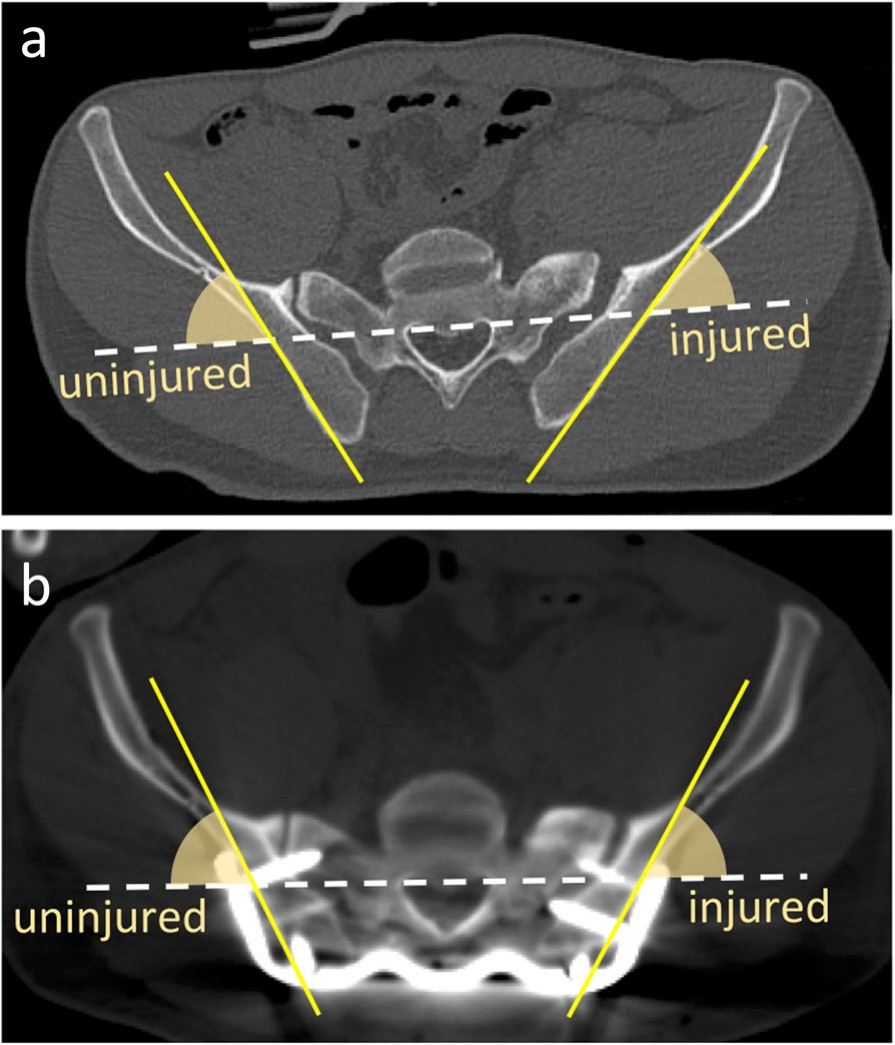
a Pre- and b Post-operative CT evaluation. The angle between the horizontal plane of the sacrum (white dotted line) and the lateral surface of the ilium (yellow line) were measured bilaterally
